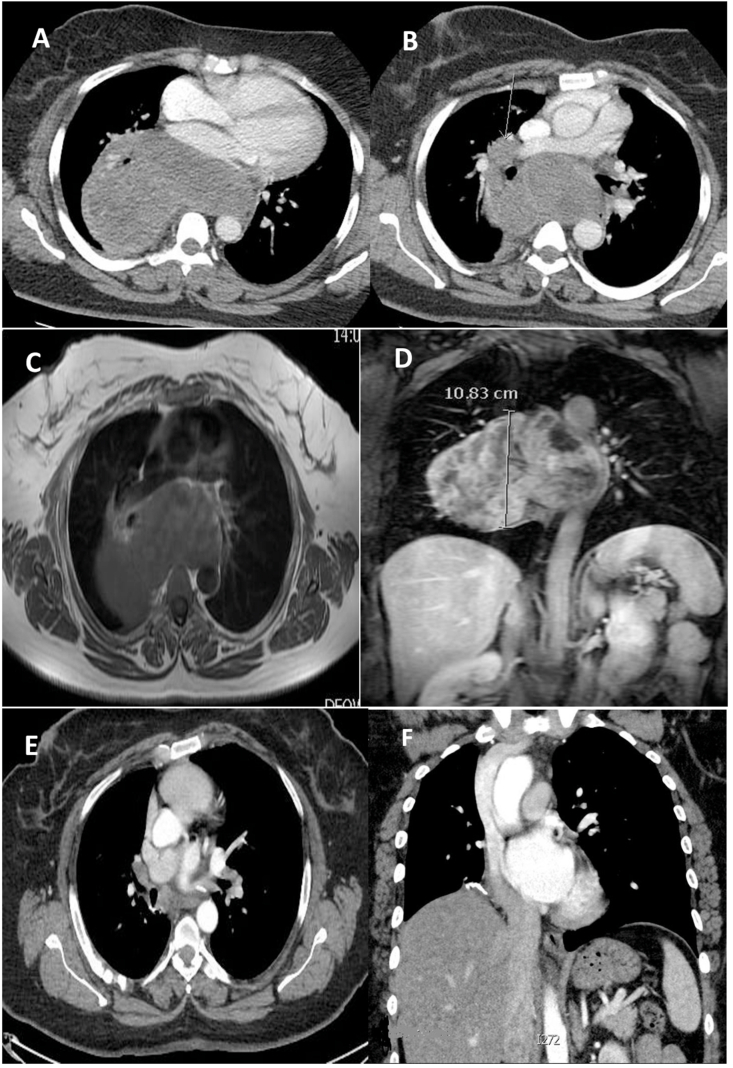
(A&B) A computed Tomographic scan of Thorax showed a large mass in the right hemithorax MRI (C&D) showing a large posterior mediastinal mass and it relation with vascular structures. (E&F) Post operative CT scan of Thorax 14 moths after surgery showing no recurrence.
